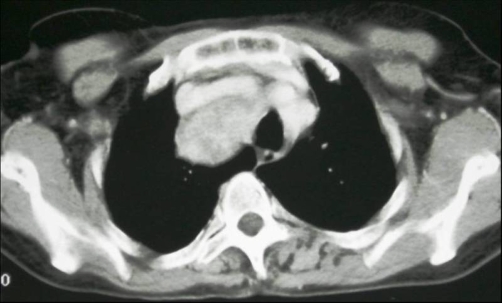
Computed tomography shows a well-circumscribed heterogeneous tumor in the right superior mediastinum.
